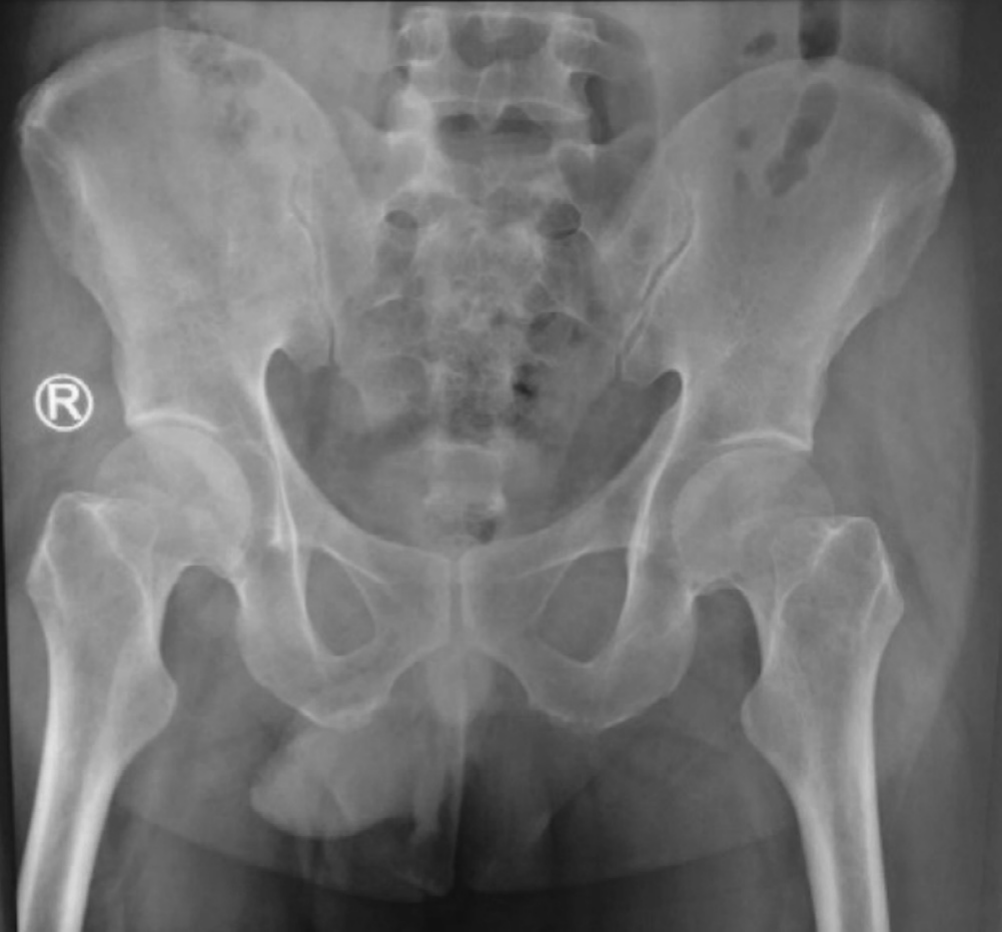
Plain abdominal X-Ray with a posteroanterior view and visible penis showed normal findings
